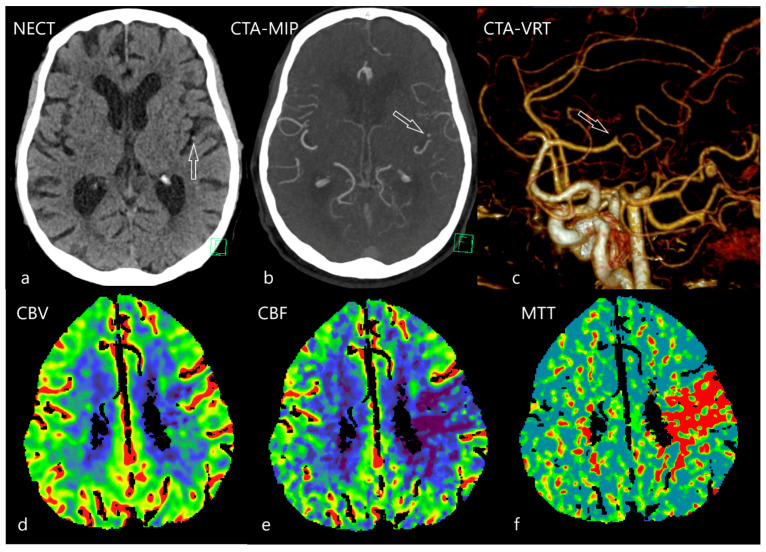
Distal medial cerebral artery occlusion by calcified embolus from mitral valve prosthesis endocarditis. 82-year-old male after mitral and tricuspid valves surgical replacement 11 years ago was admitted to emergency after the fall due to unconsciousness. He suffered from aphasia and the right sided hemiparesis; atrial fibrillation was revealed as well. On NECT (a) the small calcified dot was depicted in the Sylvian area (arrow), the same finding (arrow) was apparent on CTA with transversal maximum intensity projection (MIP) 3D reconstruction (b), the amputation of one of the arterial branches is seen on 3D volume rendering reconstruction (VRT) [(c), arrow]. On CTP (lower row) a region of hypoperfusion is present (e), with prolonged mean transit time (MTT) [red area, (f)] and decreased cerebral blood flow (CBF) [blue area, (e)]; however, the cerebral blood volume (CBV) was not affected [blue area, (d)]. Mitral valve prosthesis endocarditis was revealed as the probable source of emboli.
